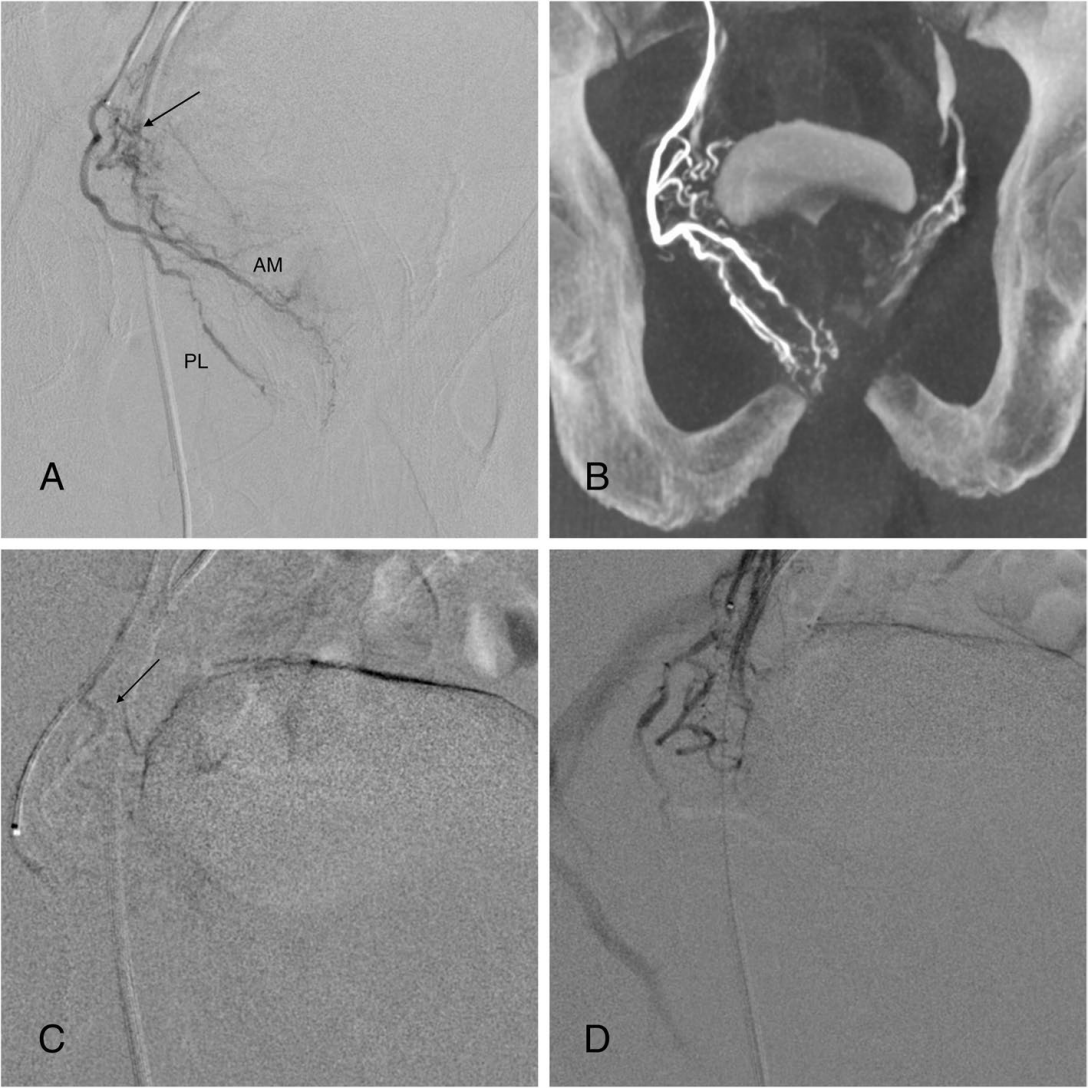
A Selective right prostatic artery DSA in oblique view showing the normal posterolateral (PL) and anteromedial (AM) prostatic branches. Cranially, a large branch to the bladder was also observed (arrow). B Intraprocedural Cone Beam CT in coronal view confirming the findings seen in DSA. C DSA while microspheres were injected demonstrating reflux to the bladder branch (arrow). D Final DSA after embolization showing devascularization of the prostatic branches and preservation of the bladder arterial branch
